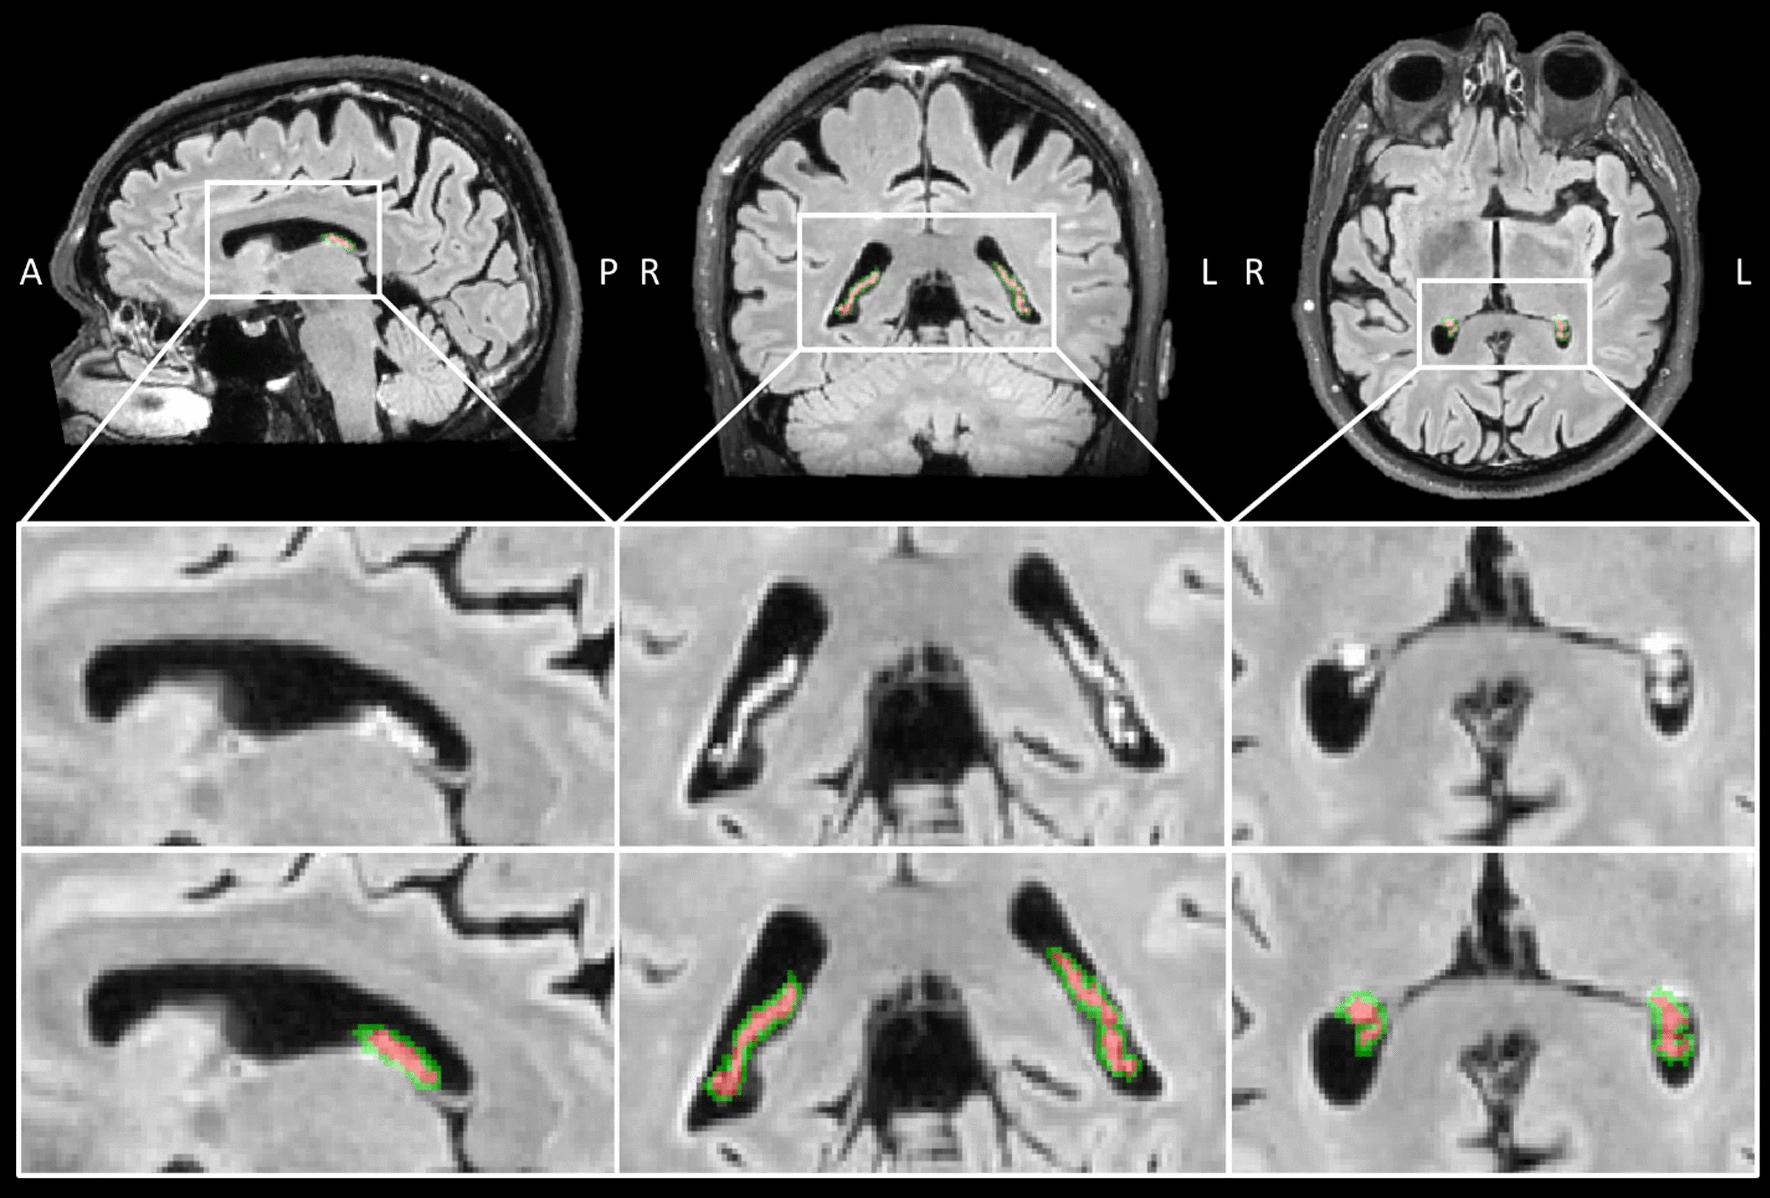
Representative manual segmentation of the choroid plexus (CP, red), and the transition area between the choroid plexus and CSF (green) identified on the post-GBCA FLAIR MRI images (69 years old, male). The regions in the white boxes on the first row are magnified on the second and third rows. The images on the second and third rows are identical with the CP and transition area outlined with red and green, respectively on the third-row images. A: anterior; P: posterior; R: right; L: left. FLAIR: fluid-attenuated inversion recovery; GBCA: gadolinium-based-contrast-agent; CSF: cerebrospinal fluid
